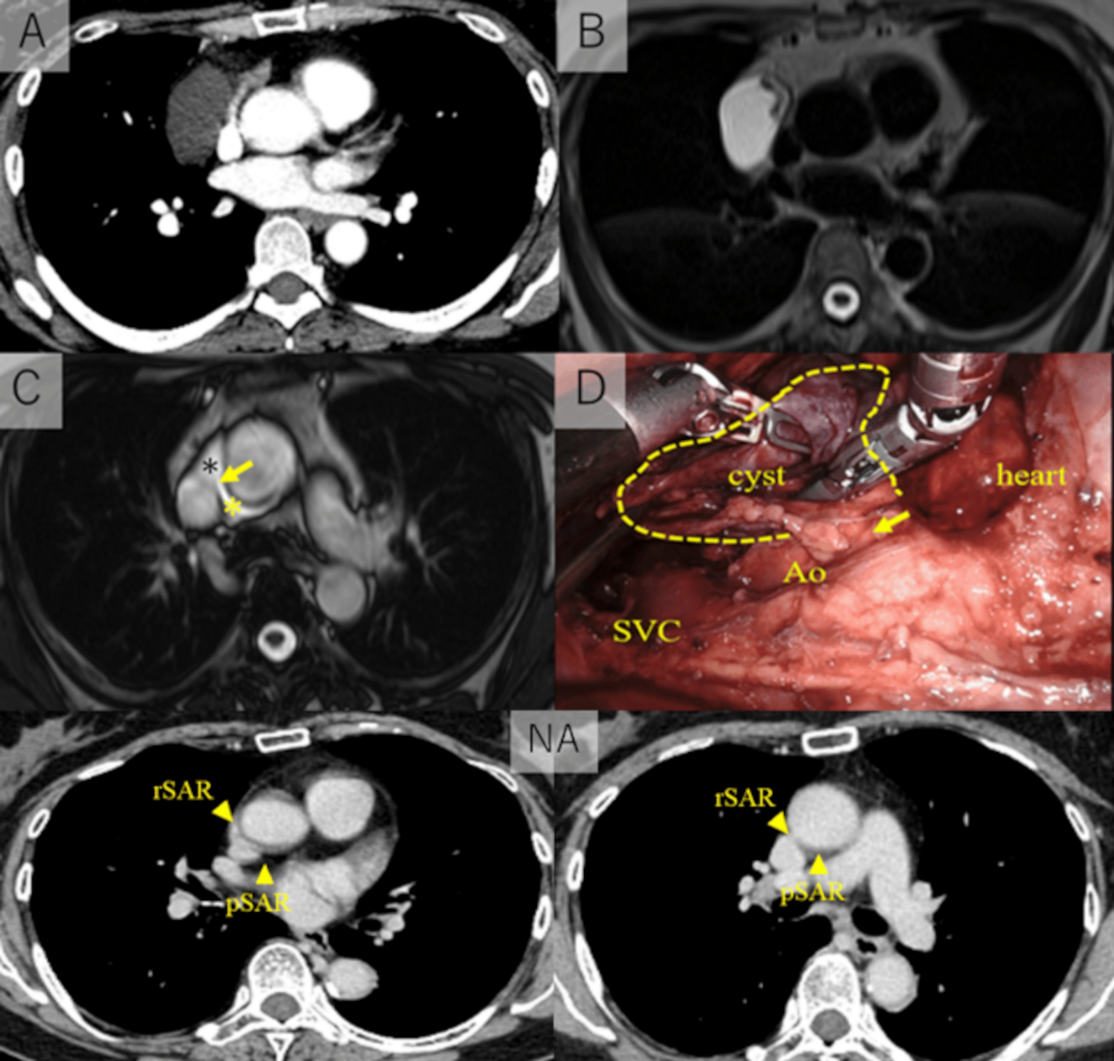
Radiological images and the operative findings of Case 2 A: Computed tomography revealed a fluid density mass (5.1 x 2.9 cm) in thymus. B: Magnetic resonance image (MRI) of the mass showed a high density on T2-weighted imaging. C: The origin of the lesion was a fluid collection of the right lateral portion of the superior aortic recess (black *). The fluid collection is connected to the fluid collection of the posterior portion of the superior aortic recess (yellow *) through the yellow arrow route. D: The cystic tumor is connected to the space in front of the aorta (yellow arrow = connected part). NA: The normal anatomy figure (reference). Yellow arrowheads are in the pSAR and the rSAR. SVC = superior vena cava; Ao = aorta; pSAR = posterior portion of the superior aortic recess; rSAR = right lateral portion of the superior aortic recess.
